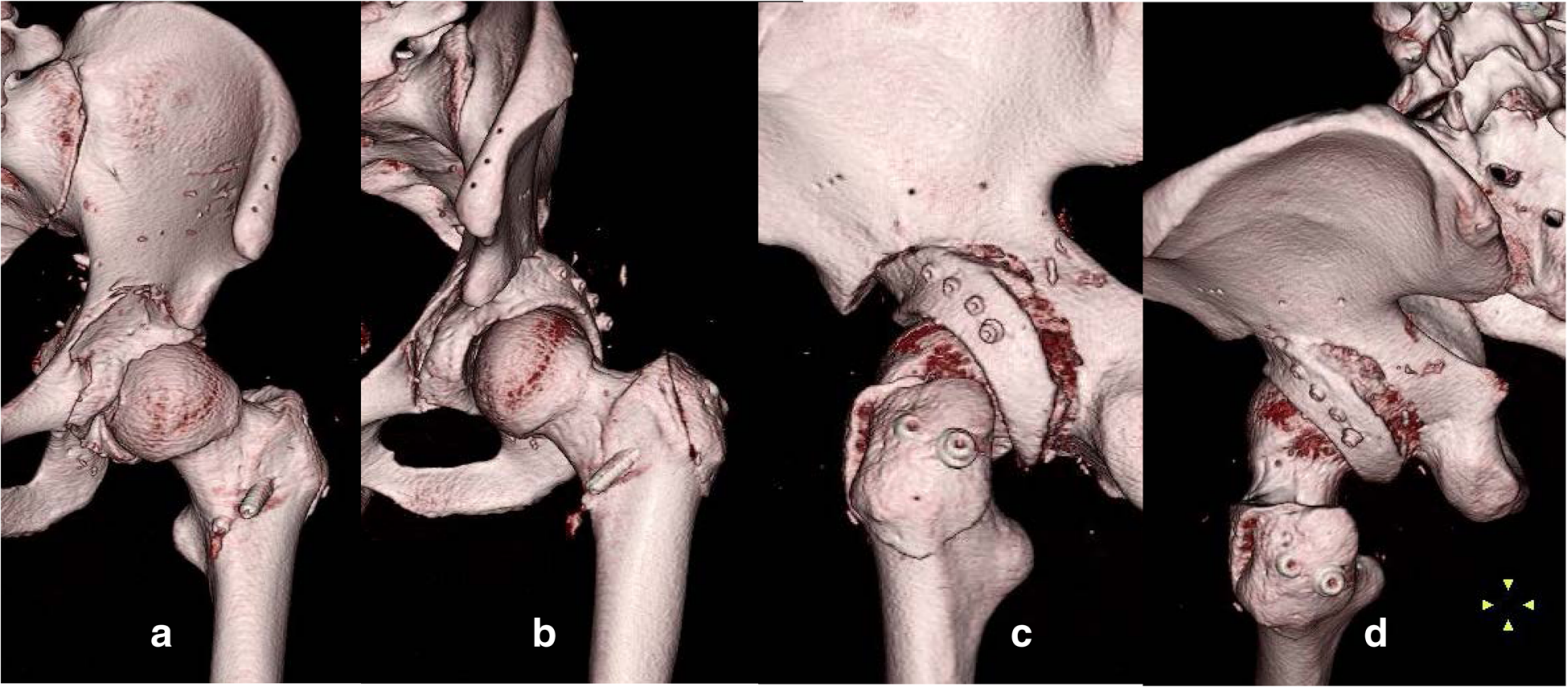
We performed three-dimensional computed tomography 2 weeks postoperatively. a–d Postoperative three-dimensional model of the pelvis
